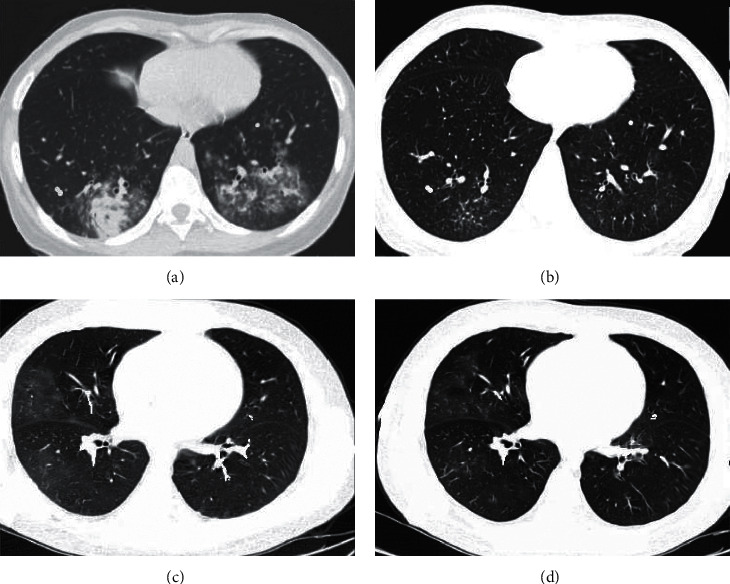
CT image characteristics of children before and after treatment.
